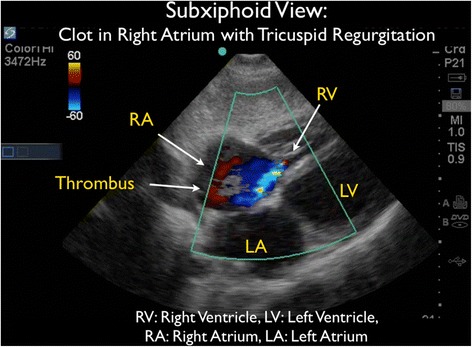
Doppler US interrogation of the tricuspid valve taken from the subxiphoid echo view. Significant tricuspid regurgitation is present and this blood flow moves the thrombi retrograde into the right atrium from the right ventricle. LA, left atrium; LV, left ventricle; RA, right atrium; RV, right ventricle.
